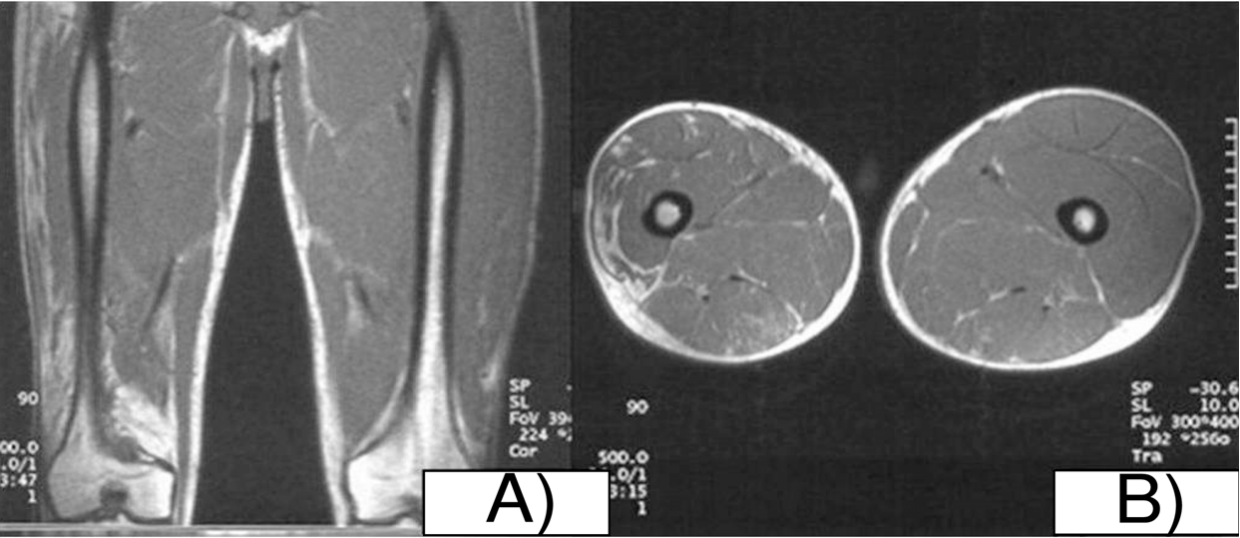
Coronal and axial magnetic resonance imaging of patient 1. His left extremity was thicker than his right extremity, as shown on coronal (A) and axial (B) images.
